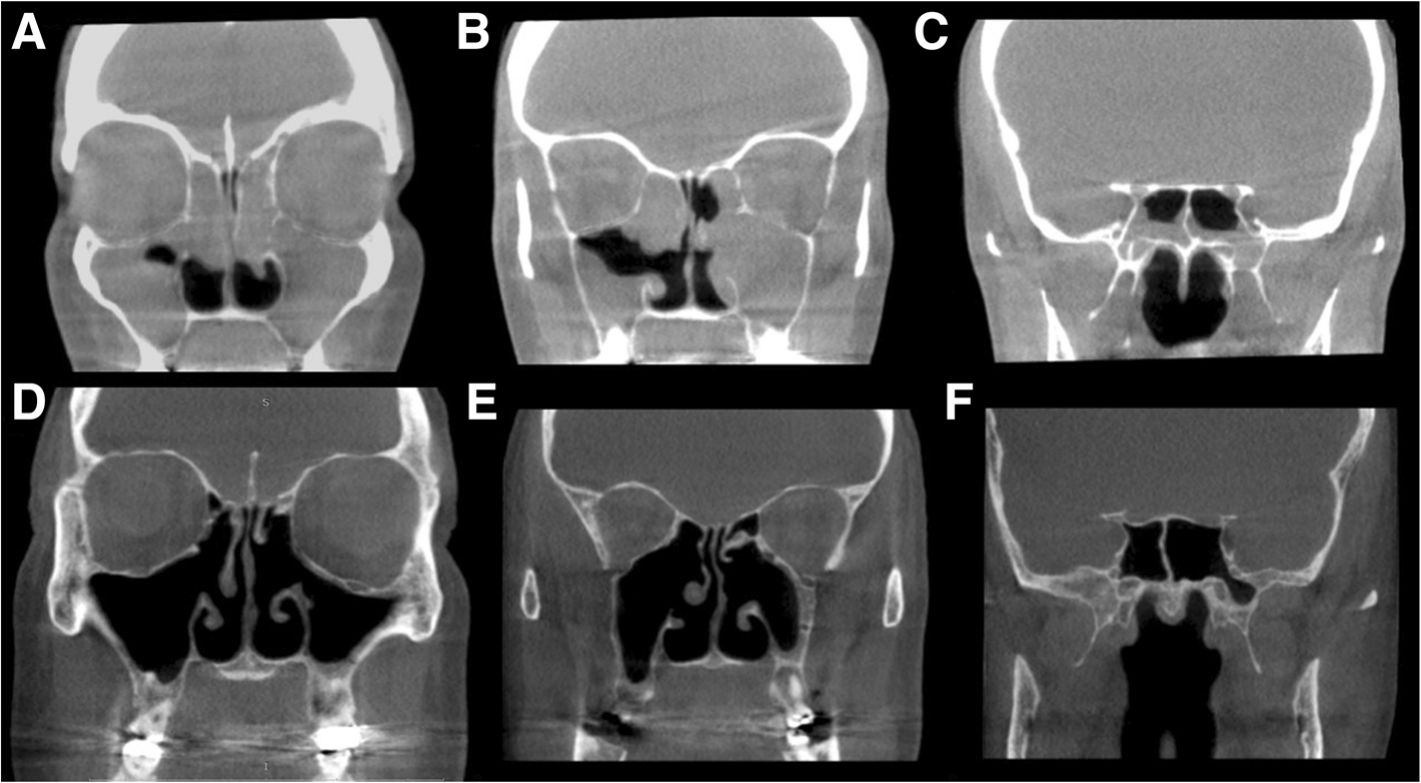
CT images of patient suffering from non-eCRS airway inflammation compliant on topical therapy; a-c: Post-operative imaging, with persistent disease; d-f: After initiation of macrolide therapy for 3 months (Clarithromycin 250 mg daily)
